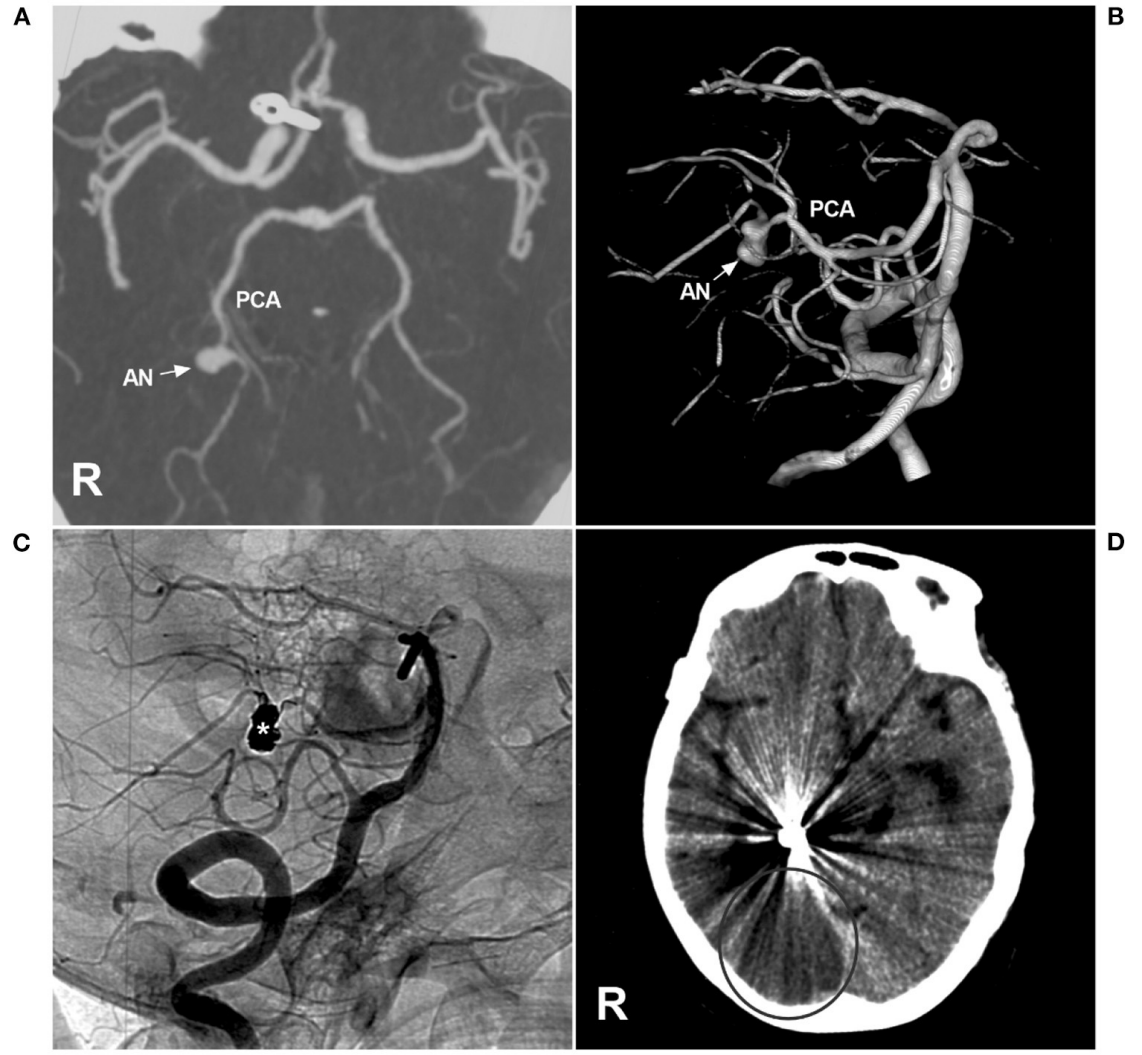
Brain infarction after PAO for P3 segment aneurysms. (A,B) CTA (A) and three-dimensional angiogram (B) showing the aneurysm (arrow and AN) on the right P3 segment. (C) The aneurysm (asterisk) was treated by coiling PAO. (D) Postoperative CT showing infarction in the right occipital lobe. AN, aneurysm; CT, computed tomography; CTA, CT angiography; PCA, posterior cerebellar artery; R, right; VA, vertebral artery.
